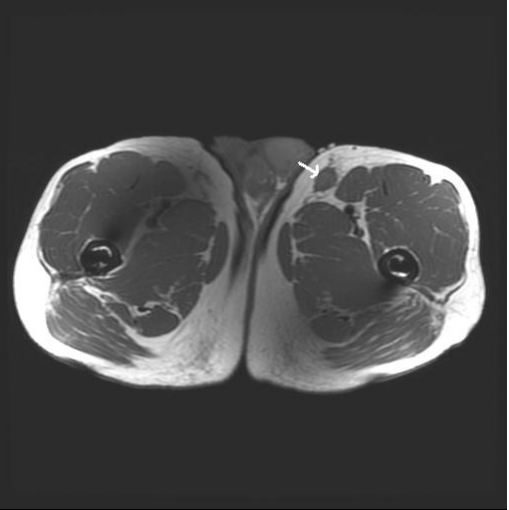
MRI shows a solid, vascular enhancing subcutaneous thigh mass with spiculated margins.(see arrow)
